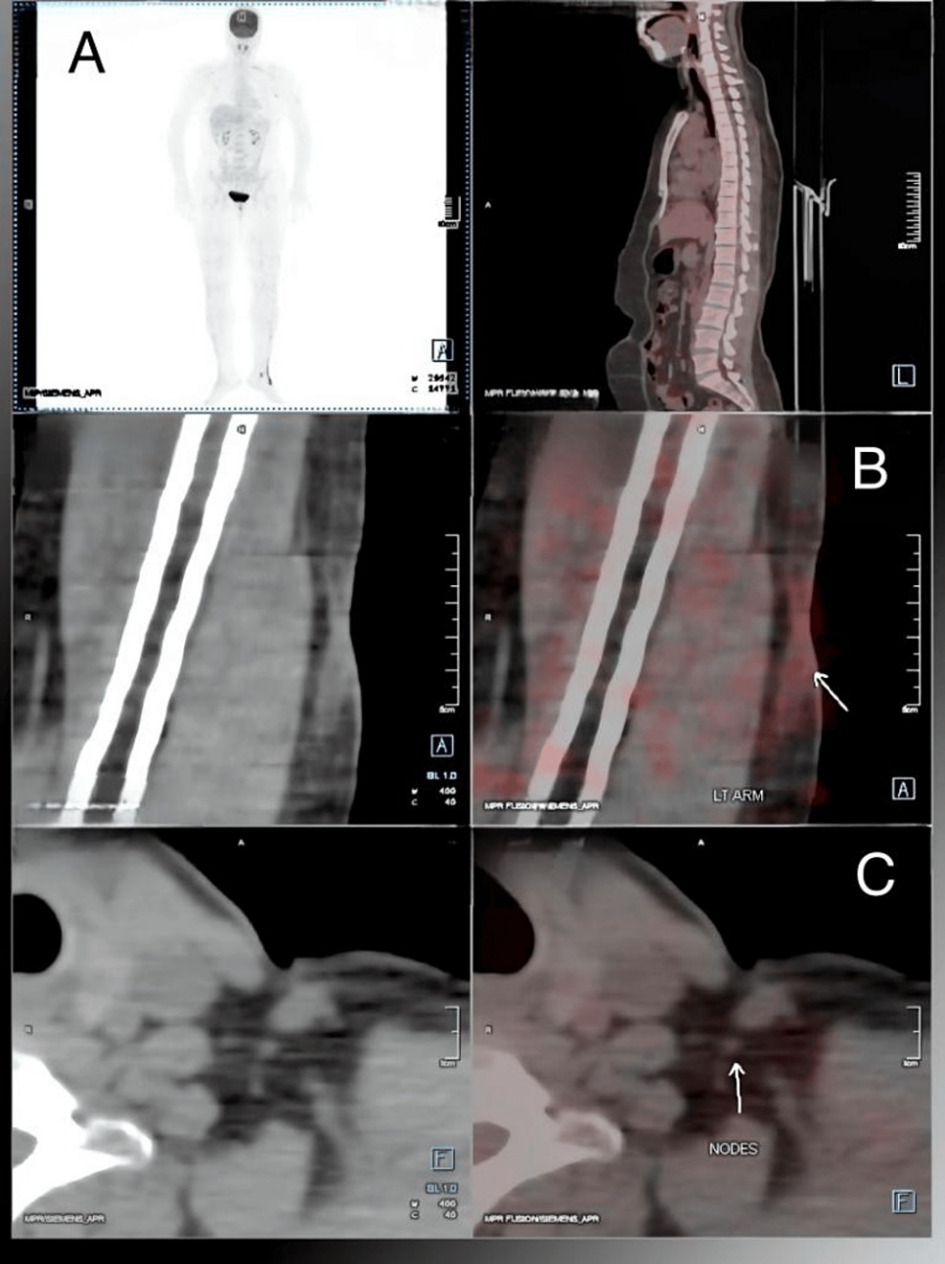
Positron emission tomography-computed tomography scan. (A) Physiological uptake of the tracer. (B) Weakly metabolic cutaneous-subcutaneous thickening in the outer aspect of the left arm. (C) Ametabolic left axillary nodes.
